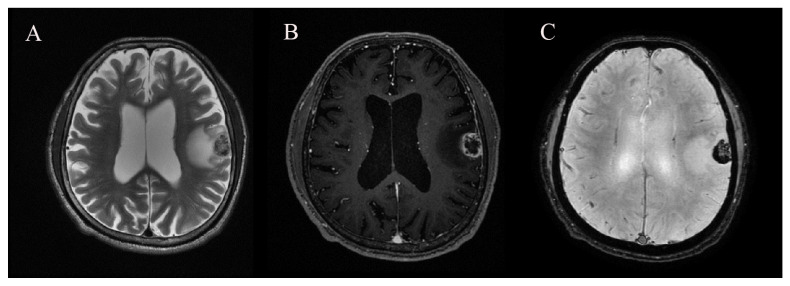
A 67-year-old man with hemorrhagic brain metastasis (BM) in left frontoparietal lobe. (A) T2-weighted imaging, (B) contrast-enhanced 3D T1-weighted imaging, and (C) susceptibility-weighted angiography (SWAN).
